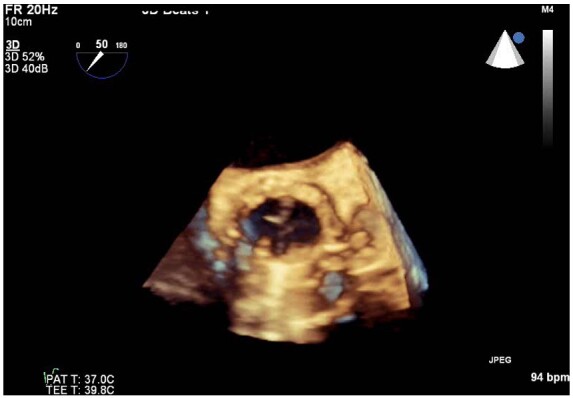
Three-dimensional transoesophageal echocardiogram of short-axis view with showing peri-annular aortic root abscess.
